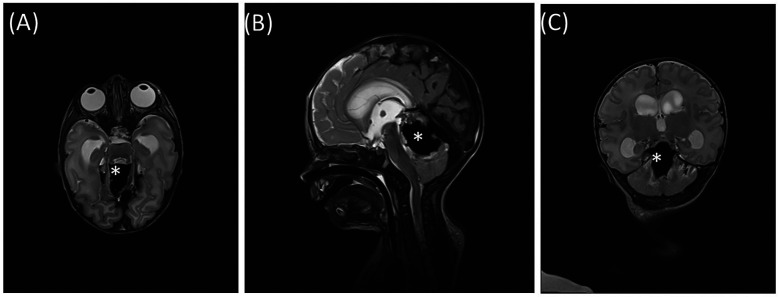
T2—MRI before surgery (A) axial, (B) sagittal, (C) coronal. * Hematoma.
